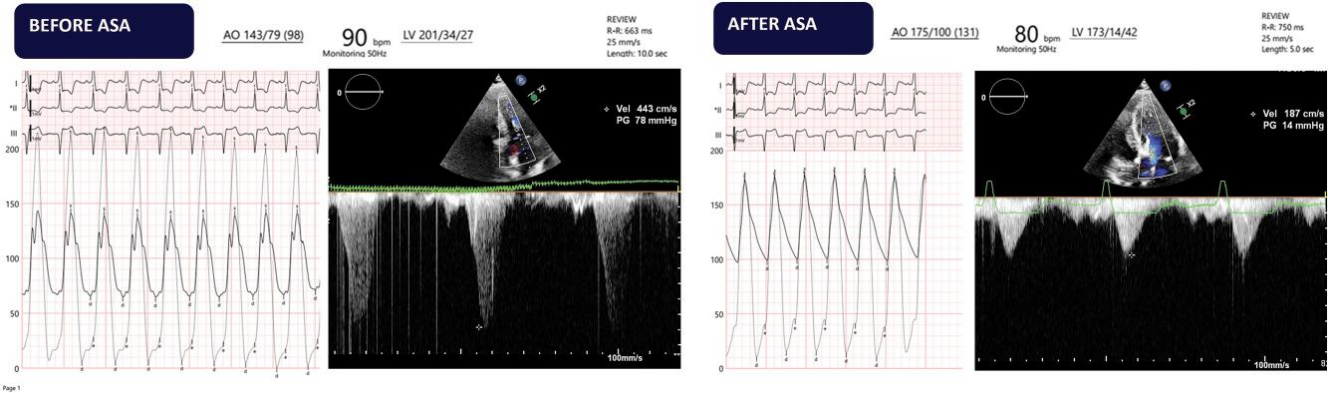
Intra-procedural aspects: pressure curves measured trough hear catheterization and left ventricular outflow tract gradient measured trough Doppler echocardiography before and after alcohol septal ablation, showing significant reduction in gradient.
